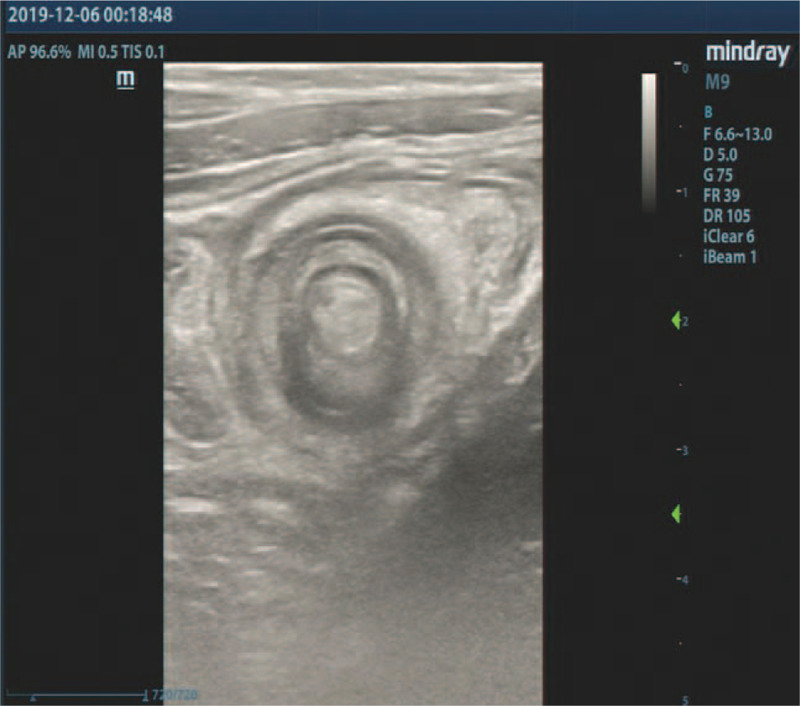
Axial sonogram of the intussusception shows a multiconcentric ring sign with a multilayer appearance.
